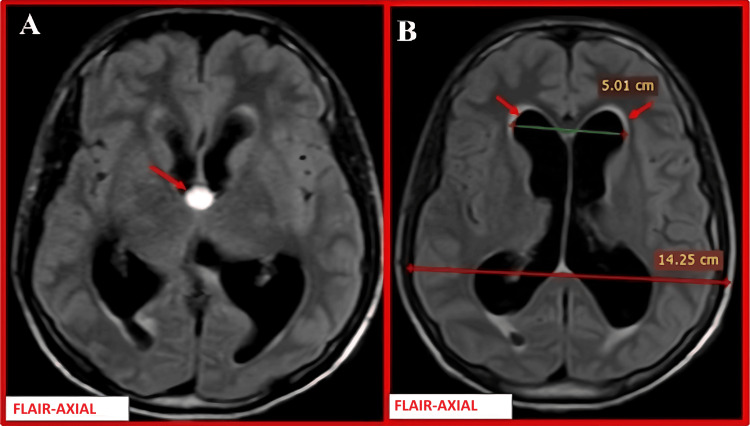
MRI brain images in (A and B) axial FLAIR sections (A) Axial FLAIR image shows a hyperintense cystic lesion at the roof of the third ventricle (red arrow), measuring approximately 12 × 11 × 14 mm, obstructing the foramen of Monro. (B) Axial T2-weighted image demonstrates dilated bilateral lateral ventricles (red arrows) with an Evans index of 0.35, consistent with acute obstructive hydrocephalus. Note: All images are original and obtained from the institutional study dataset. FLAIR, fluid-attenuated inversion recovery
